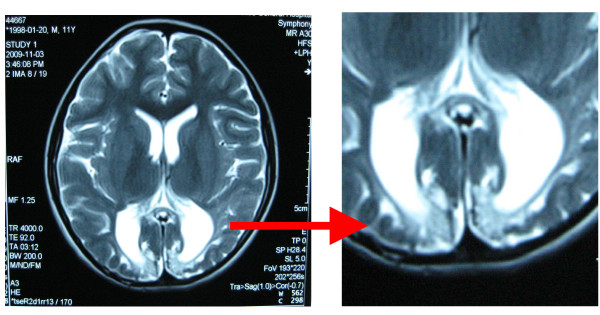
Brain MRI showed the bilateral parietal-occipital lobe and corpus callosum dysplasia.
